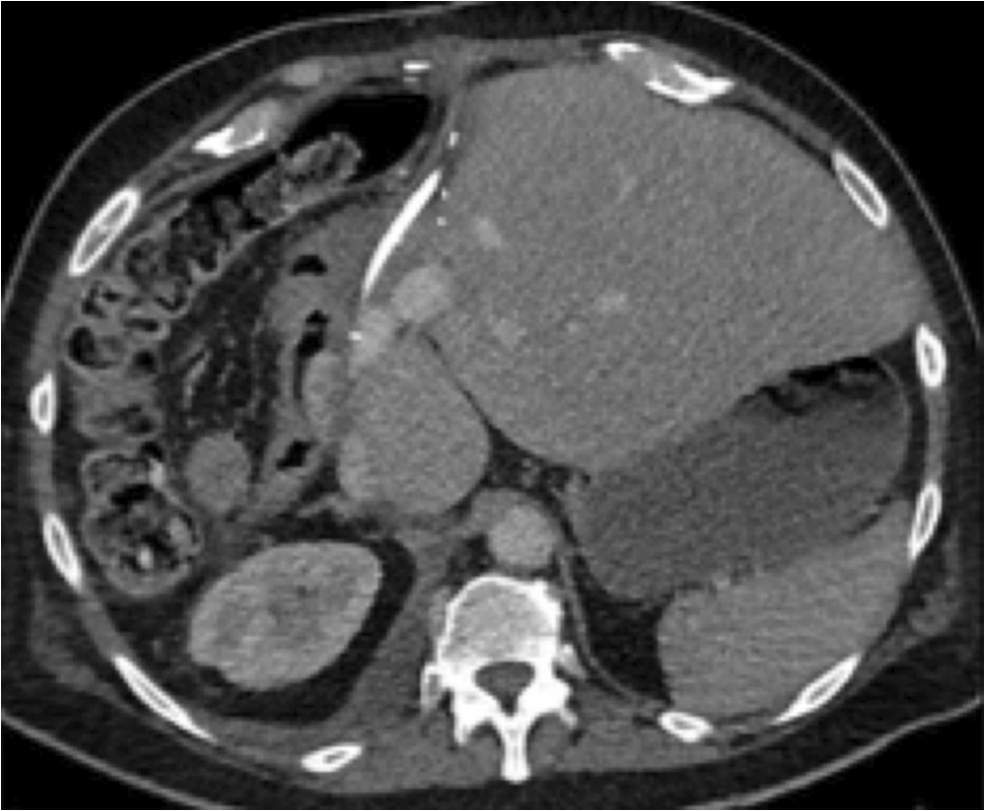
Contrast-enhanced CT following extended right hepatectomy with roux-en-y hepaticojejunostomy
